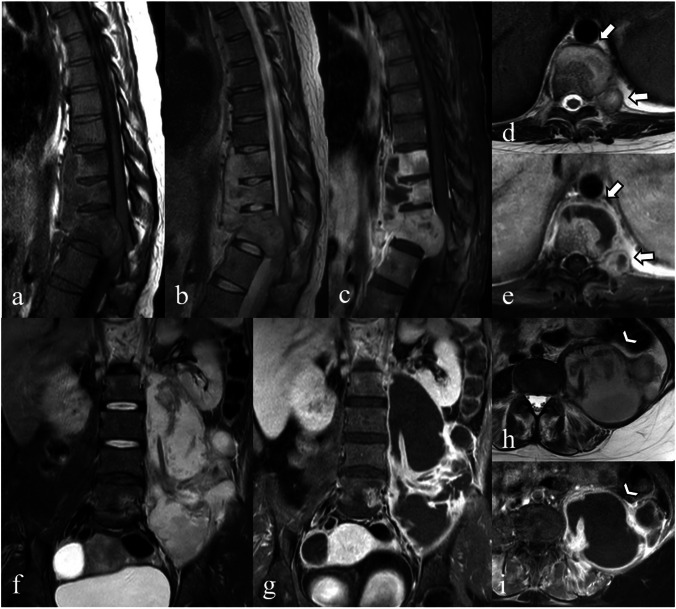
Magnetic resonance images of a 29-year-old female patient with tuberculous spondylitis. Sagittal T1-weighted (a), T2-weighted (b), and fat-suppressed contrast-enhanced T1-weighted (c) images show contiguous destruction of T9–T12 vertebrae with severely collapsed T11–T12 vertebral bodies causing severe kyphotic angulation (gibbus deformity). Anterior subligamentous spreading, intraosseous abscess, and epidural abscess with conus medullaris compression are also noted. Axial T2-weighted (d) and fat-suppressed contrast-enhanced T1-weighted (e) images show intraosseous and left paravertebral abscesses (arrows in d and e). Coronal T2-weighted (f), fat-suppressed contrast-enhanced T1-weighted (g), axial T2-weighted (h), and fat-suppressed contrast-enhanced T1-weighted (i) images also show large left paravertebral abscess along the left psoas muscle (psoas abscess), extending to left-sided pelvic cavity (arrowheads in h and i)
